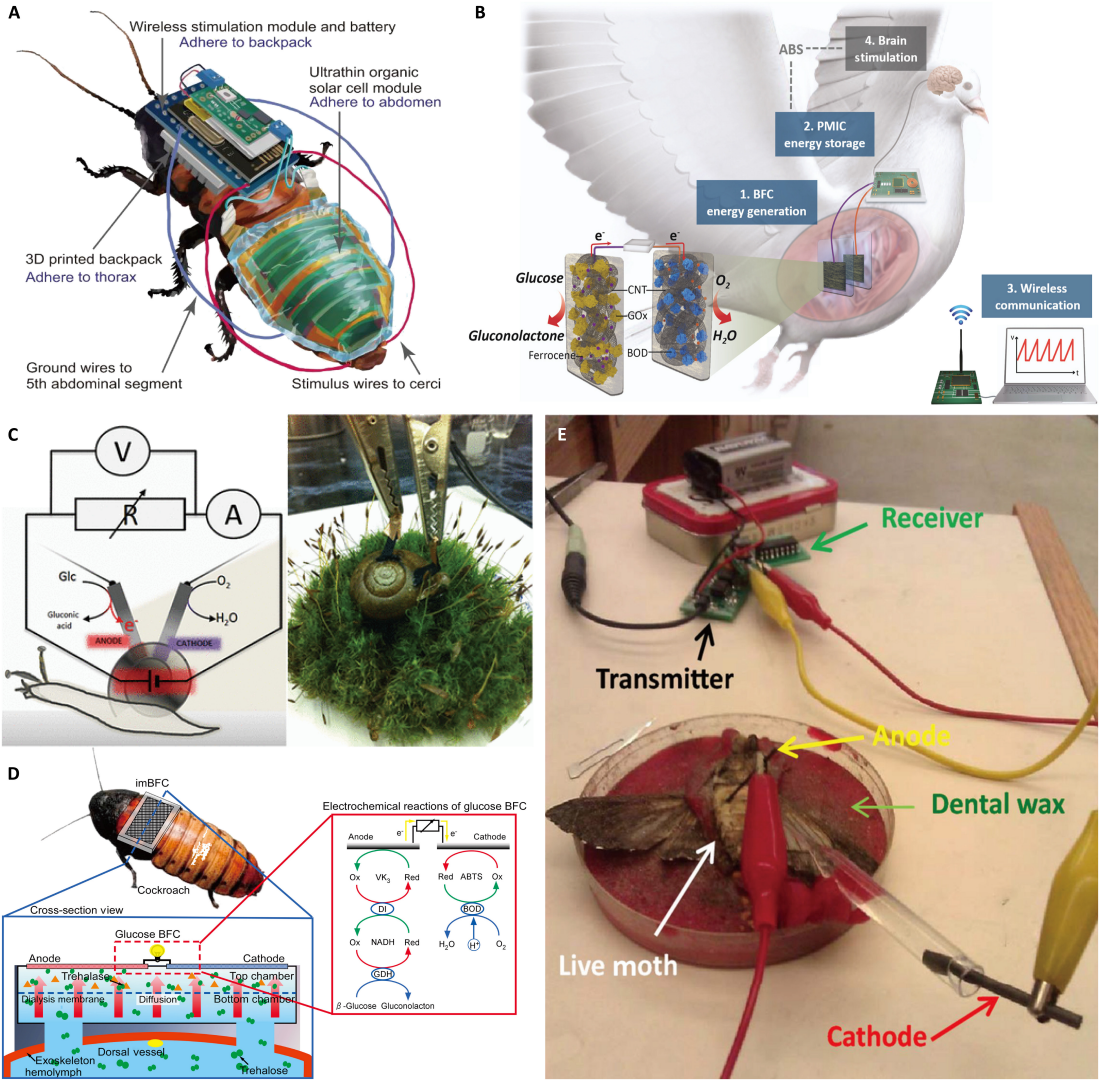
BHRs using solar and biofuel cells for energy supply. (A) Ultrasoft organic solar cell integrated on the body of a cockroach–machine hybrid robot [31]. Adapted from [31] with the permission under the terms of the CC-BY license. (B) Biofuel cell to power the pigeon–machine hybrid robot [33]. Copyright 2021 Elsevier. (C) Biofuel cell implanted in a snail [90]. Copyright 2012 American Chemical Society. (D) Enzymatic biofuel cell to power a cockroach control backpack [7]. Copyright 2016 Elsevier. (E) Biofuel cell implanted in a moth [92]. Adapted from [92] with the permission under the terms of the CC-BY license.
